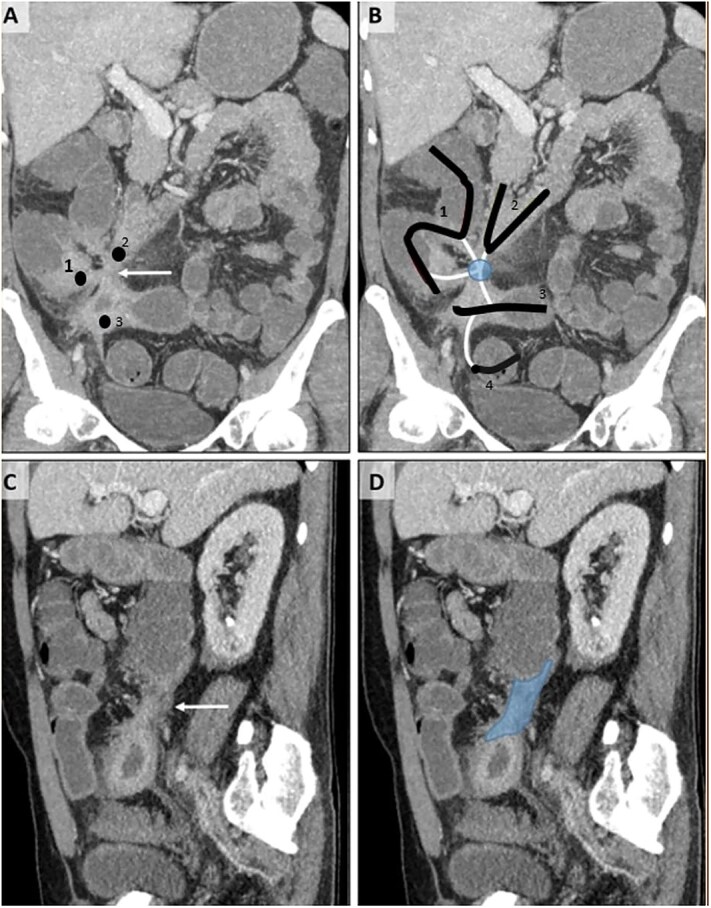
CT enterography examination, coronal (A, B) and sagittal (C, D) MIP (maximum intensity projection) images.Thickening and increased contrast enhancement are observed in the walls of the 2nd–3rd parts of the duodenum (2), terminal ileum (3), and cecum (1) (A). The relationship of these structures, whose lumens are indicated by the same numbers, with the tract-like structures (white lines) of the sigmoid colon (4) — fistulization (circle) — is shown (B). In the sagittal images (C, D), a fistula tract (D, dark area) with a thick wall (C, white arrow) is observed.
